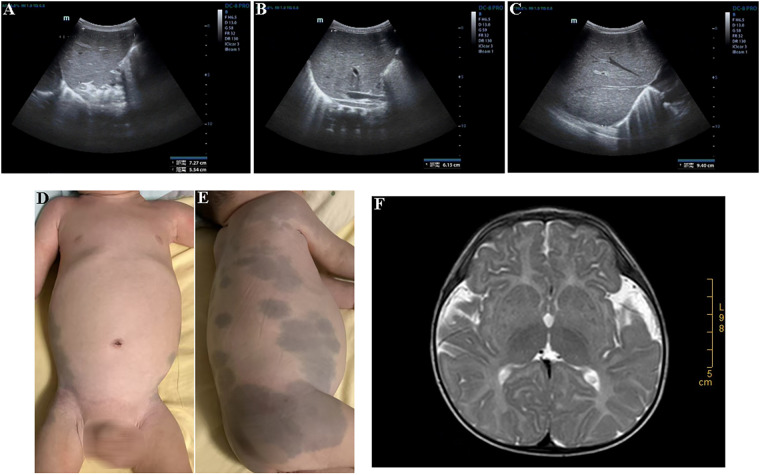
Clinical and imaging findings of the proband. (A–C) Abdominal ultrasonography at 11 months of age demonstrated hepatomegaly, indicated by measurements of the left liver lobe (longitudinal diameter 72.7 mm, anteroposterior diameter 55.4 mm), a subcostal extension of the liver edge of 61.5 mm, and a right liver oblique diameter of 94 mm. (D,E) Physical examination at 1.5 years of age revealed a protuberant abdomen and extensive slate-gray Mongolian spots located on the right lumbar-gluteal region and extending from the left lumbar-gluteal region to the upper thigh. (F) Brain MRI at 9 months of age revealed delayed myelination.
